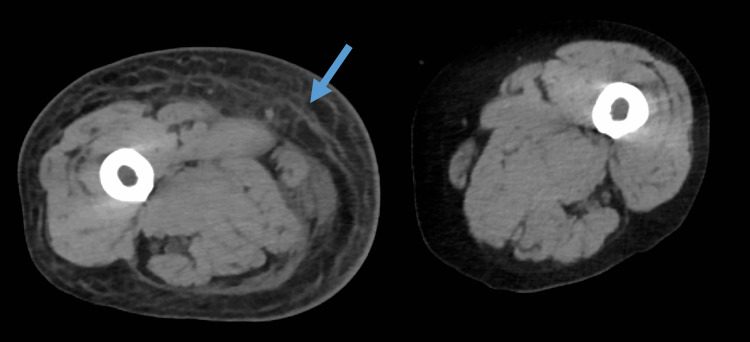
CT on arrival at hospital CT shows fascial thickening and deep soft-tissue edema with fluid tracking along fascial planes in the right medial thigh, without subcutaneous emphysema or gas formation.
